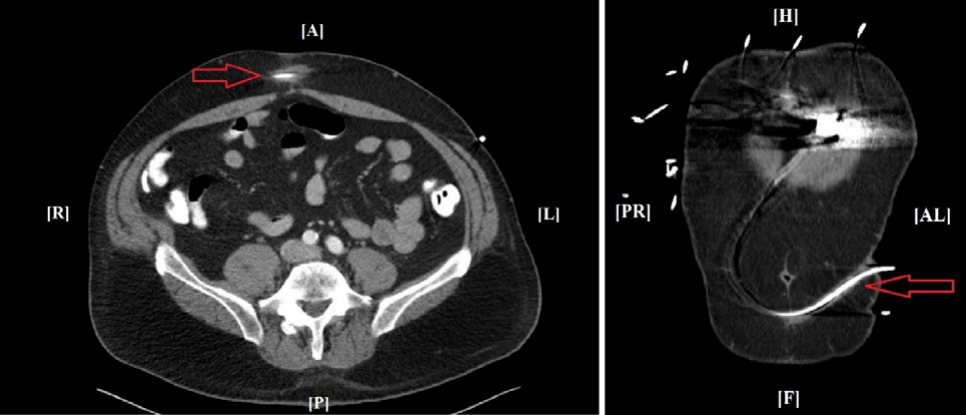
Figure 2. CT scan of the abdomen revealed soft tissue enhancement (red arrows) around the LVAD driveline in the lower abdomen.
